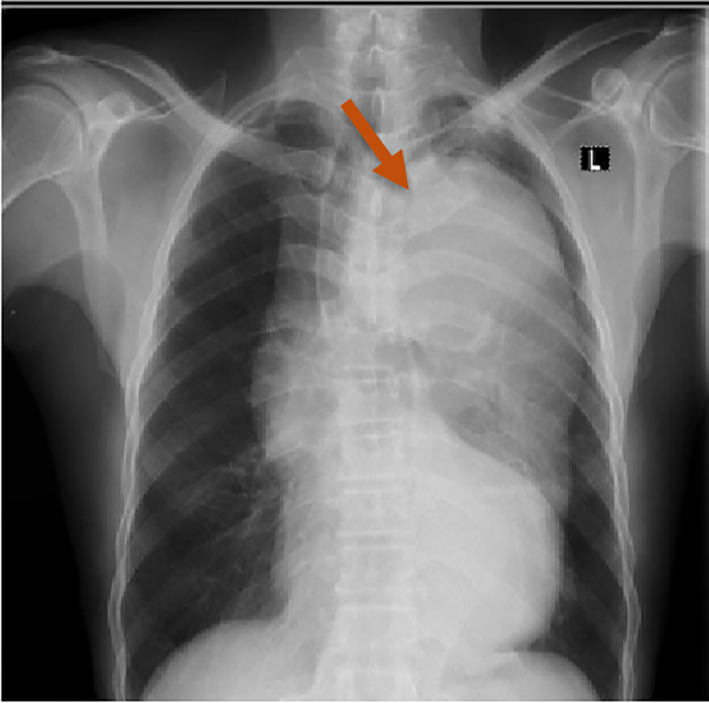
Chest radiograph showing widened mediastinum and prominent aortic shadowing.
